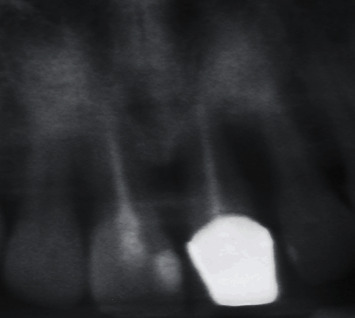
Section from panoramic radiograph representing the site of chief complaint, prior to the attempted extraction of the left maxillary central incisor. Severe vertical bone defect around the left maxillary central incisor is evident.
